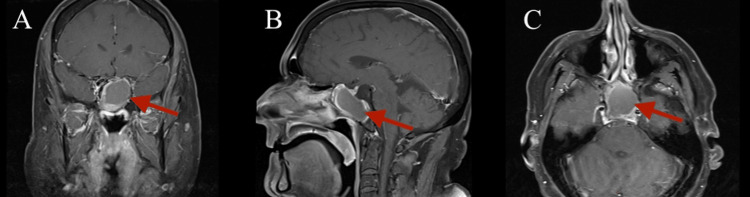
Magnetic resonance imaging (MRI) of the face shows a large T1 and T2 slightly hyperintense expansile mass in the sphenoid sinuses with extension into the suprasellar cistern (red arrows). There is displacement of the optic chiasm and pituitary stalk. A. Coronal; B. Sagittal; C. Axial
